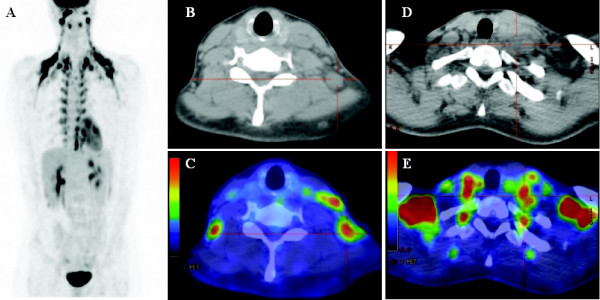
Pretreatment ^18^F-fluorodeoxyglucose (^18^F-FDG) positron emission tomography/computed tomography scan. (A) Prominent physiological brown fat uptake in the neck and thorax. (B and C) ^18^F-FDG-avid lymphadenopathy in the left lower cervical nodes. (D and E) ^18^F-FDG-avid lymphadenopathy in the left supraclavicular regions.
